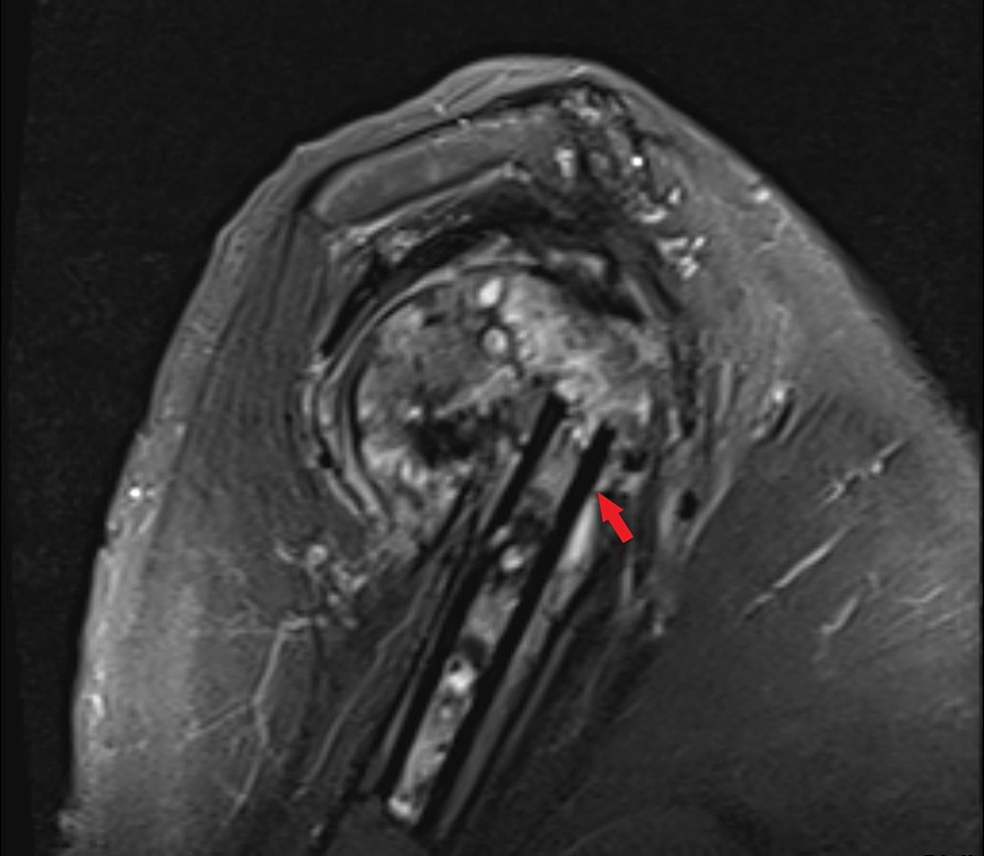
T2-weighted MRI of the proximal humerus demonstrating minimal osseous integration (red arrow).
